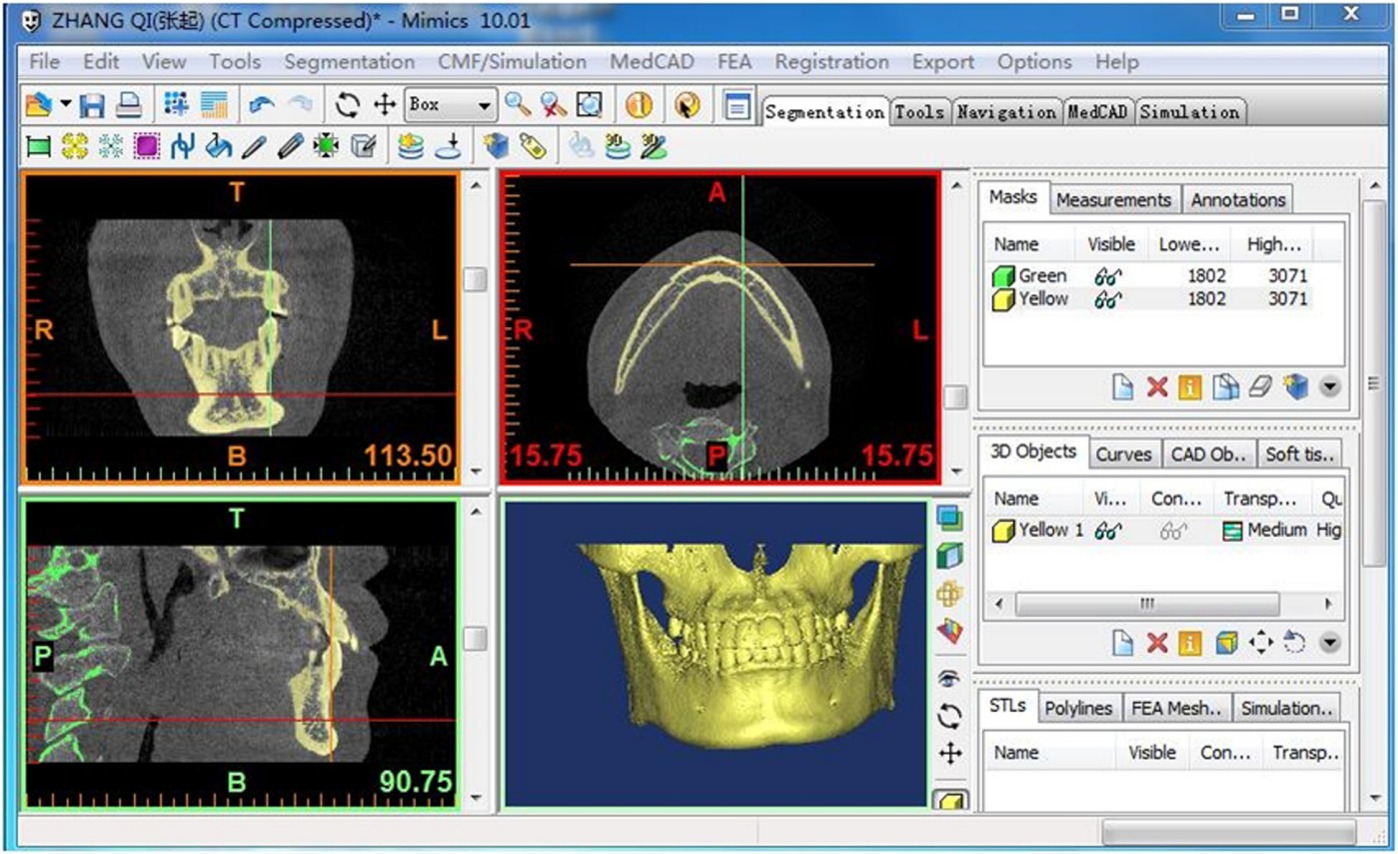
CBCT images of three slices and 3D reconstruction by Mimics
